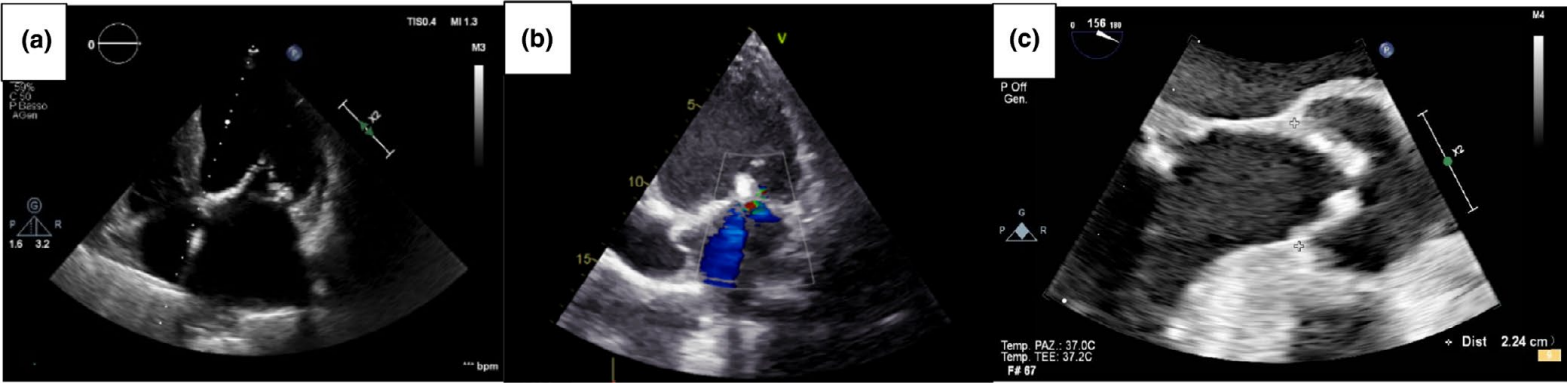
Transthoracic and transesophageal echocardiography. a–b: Apical 2 chamber view showing mitral regurgitation with calcifications of mitral leaflets and anulus (first two panels); c: Enlarged mid‐esophageal long‐axis view emphasizing aortic valve thickening, reduced systolic opening and calcifications in a CKD patient (last panel). CKD, chronic kidney disease.
